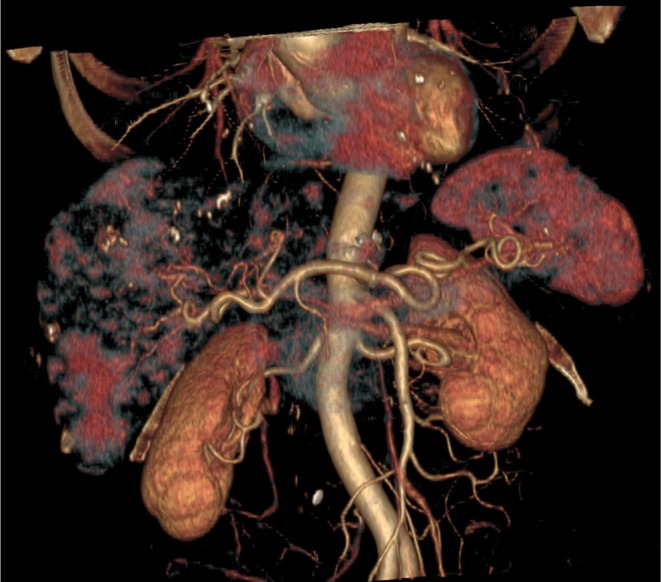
3D reconstruction of a CT scan with intravenous contrast of the upper abdomen. Large confluent vascular masses are seen in all lobes of the liver (The picture is brought with the patient's permission and an acknowledgement to Vejle Hospital Department of Radiology, which has made the reconstruction available to us).
